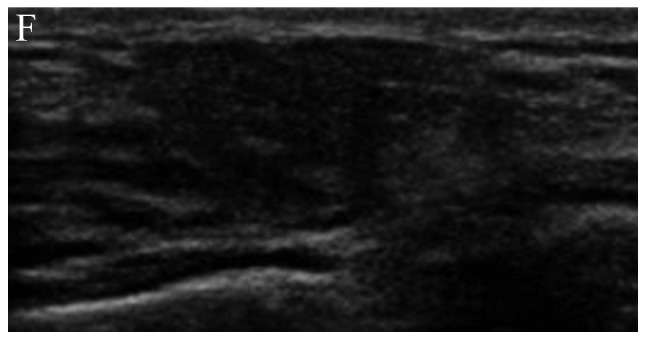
A 41-year-old woman with breast cancer. Dynamic MRI revealed an enhanced lesion that was similar in size both before NACT (A) and after the final cycle of NACT (B); the response rate was 8.3%. (C) The baseline ADC value calculated within the tumor margin was 0.789×10^−3^ mm^2^/s. (D) The %ADC after the first of four cycles of NACT was 2.3%. The response rate between before NACT (E) and after first-time NACT administration (F) was calculated as 5.9% on the ultrasound gray-scale image. NACT, neoadjuvant chemotherapy; ADC, apparent diffusion coefficient.
